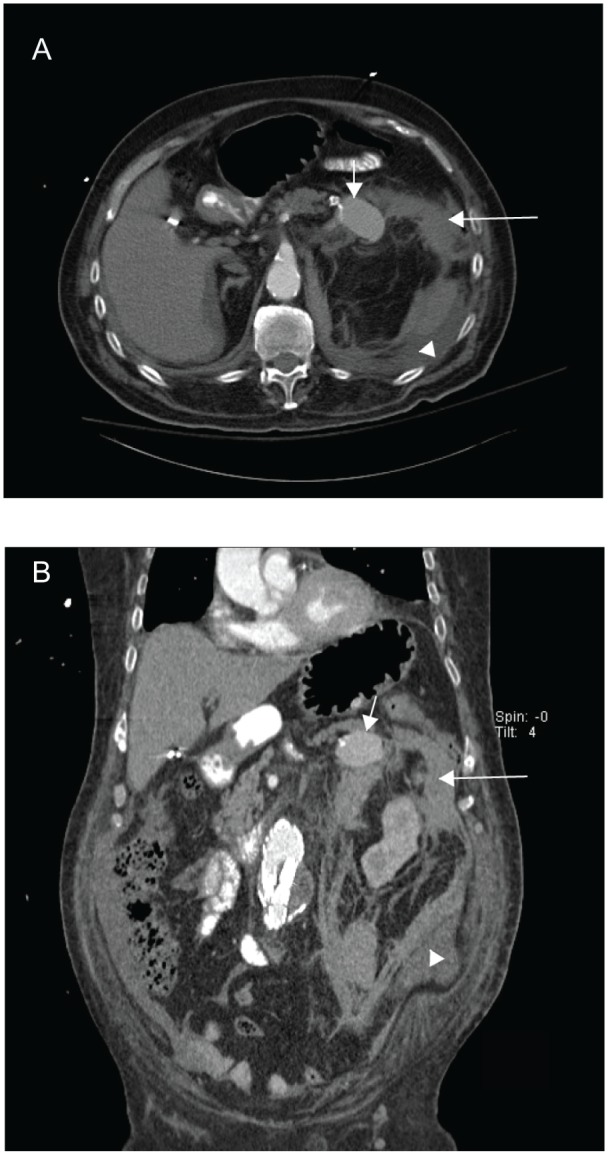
Computed tomography scan of the abdomen and pelvis. (A) An axial view of a contrast-enhanced portal venous phase CT scan demonstrates a 4.5 × 2.6 cm splenic artery pseudoaneurysm (short arrow) with associated retroperitoneal and perinephric fat stranding (long arrow). A dense abdominal free-fluid collection (arrowhead), likely from hemorrhage, is present. (B) A sagittal view of a contrast-enhanced portal venous phase CT scan demonstrates the splenic artery pseudoaneurysm (short arrow), associated retroperitoneal and perinephric fat stranding (long arrow), and the free-fluid collection (arrowhead).
